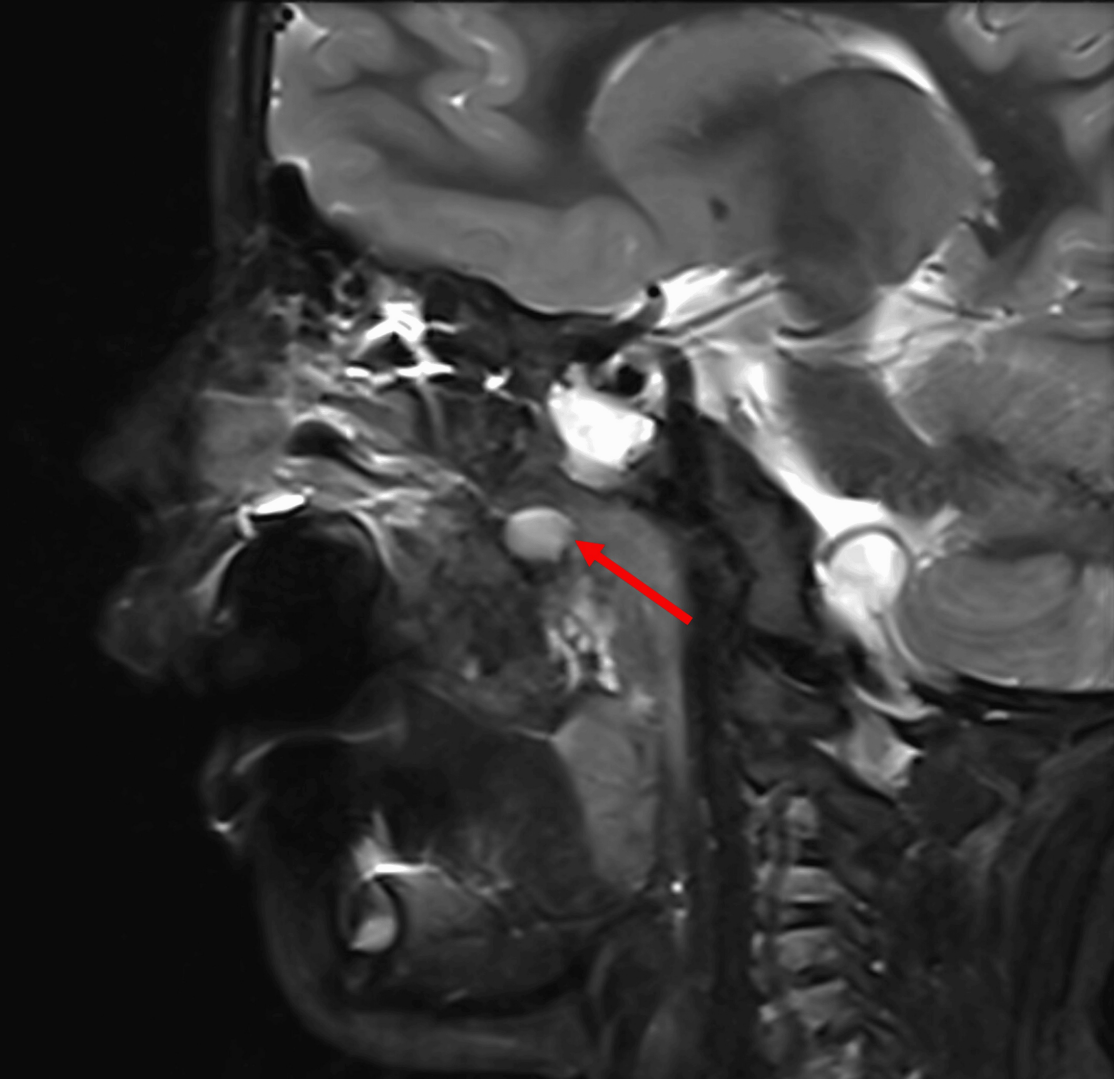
Case 2 MRI Sagittal MRI showing a well-defined oval mass at the right nasopharynx/oropharynx junction, highlighted by the red arrow.
